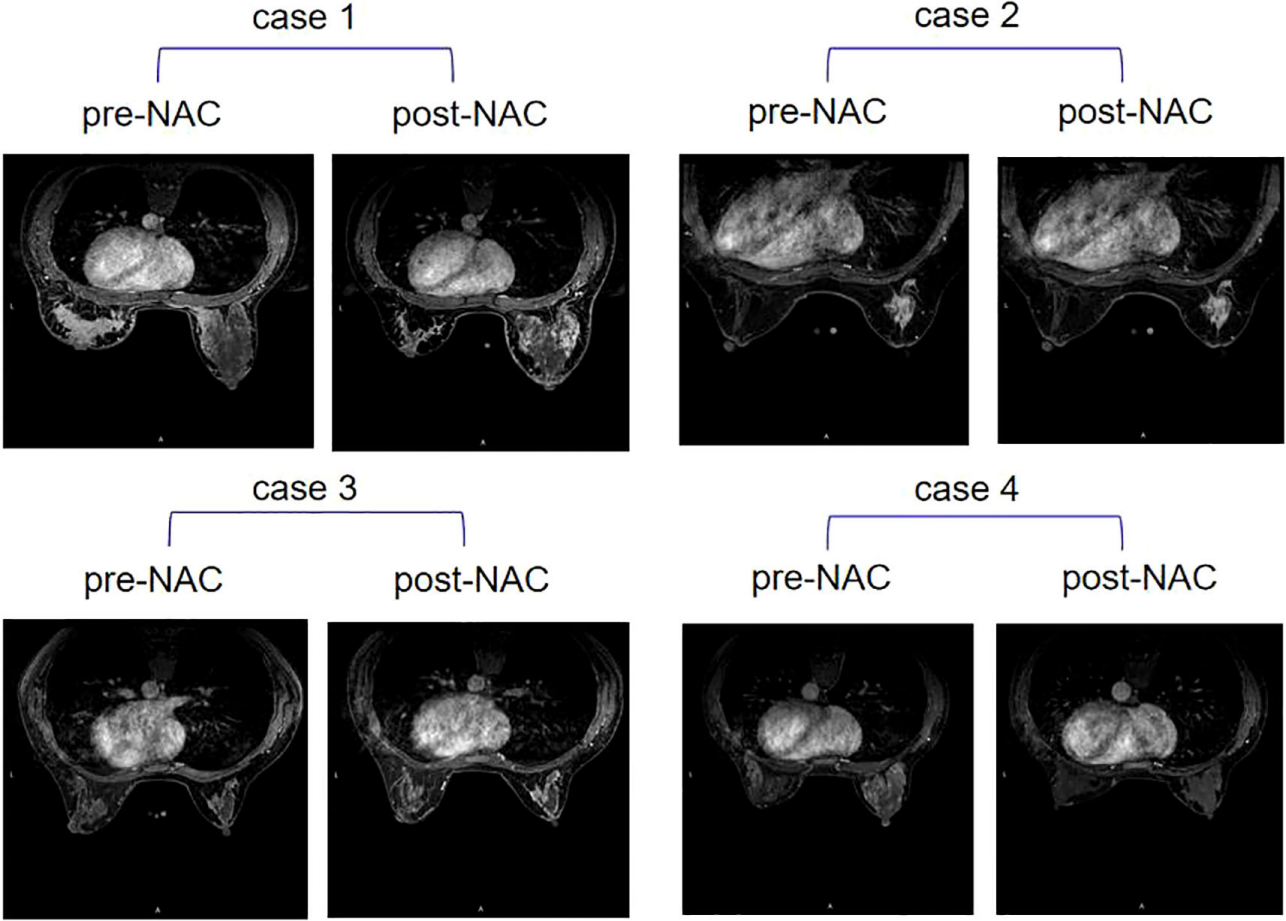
Four examples of contralateral BPE change after NAC. Case 1: BPE stayed high on pre-NAC MRI (moderate) and post-NAC MRI (moderate); case 2: BPE stayed low on pre-NAC MRI (minimum) and post-NAC MRI (minimum); case 3: BPE changed from low on pre-NAC MRI (mild) to high on post-NAC MRI (moderate); case 4: BPE changed from high on pre-NAC MRI (marked) to low on post-NAC MRI (minimum). BPE, background parenchymal enhancement; NAC, neoadjuvant chemotherapy.
